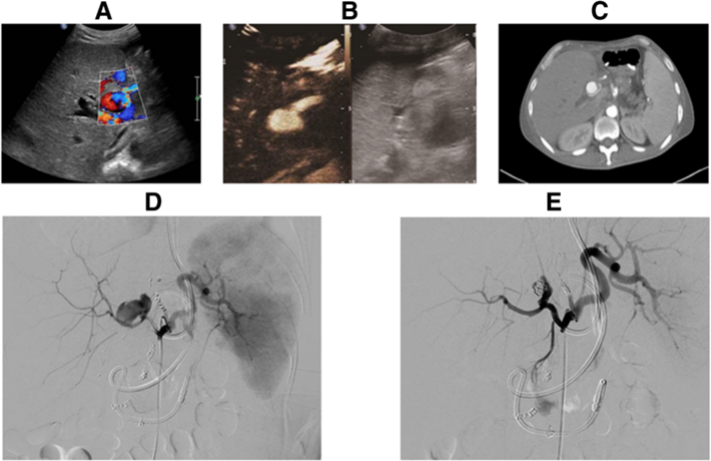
HAP was identified by (a) Color Doppler ultrasound, b CEUS and c Contrast-enhanced CT (*arrow*), and confirmed by (d) DSA. e The patient underwent coil embolization combined with thrombin injections, and the lumen of the pseudoaneurysm was totally occluded, which was confirmed by DSA
